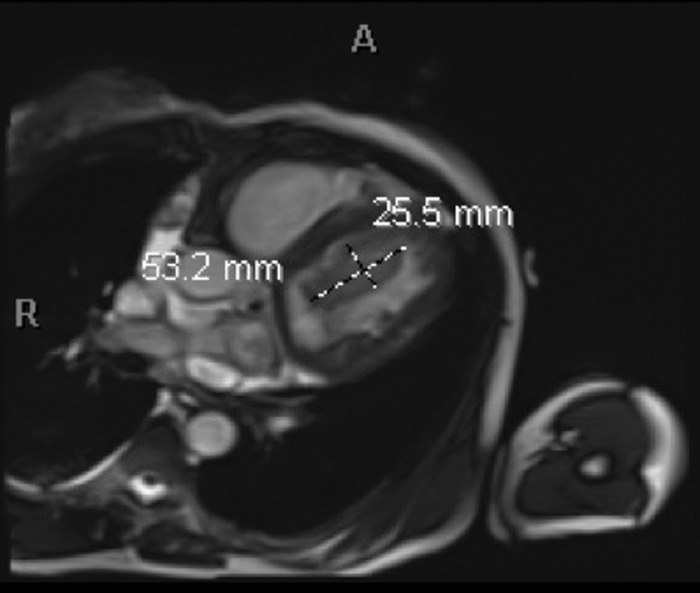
Cardiac magnetic resonance imaging with true fast imaging with steady-state free precession (TRUFI) sequence showing oblong left ventricle mass along the basal and mid anterior wall measuring approximately 5.3 mm × 2.5 mm in the 4-chamber view. The signal of this mass is slightly higher than the myocardium on the TRUFI precontrast images and is a high signal on the T2-weighted images.
